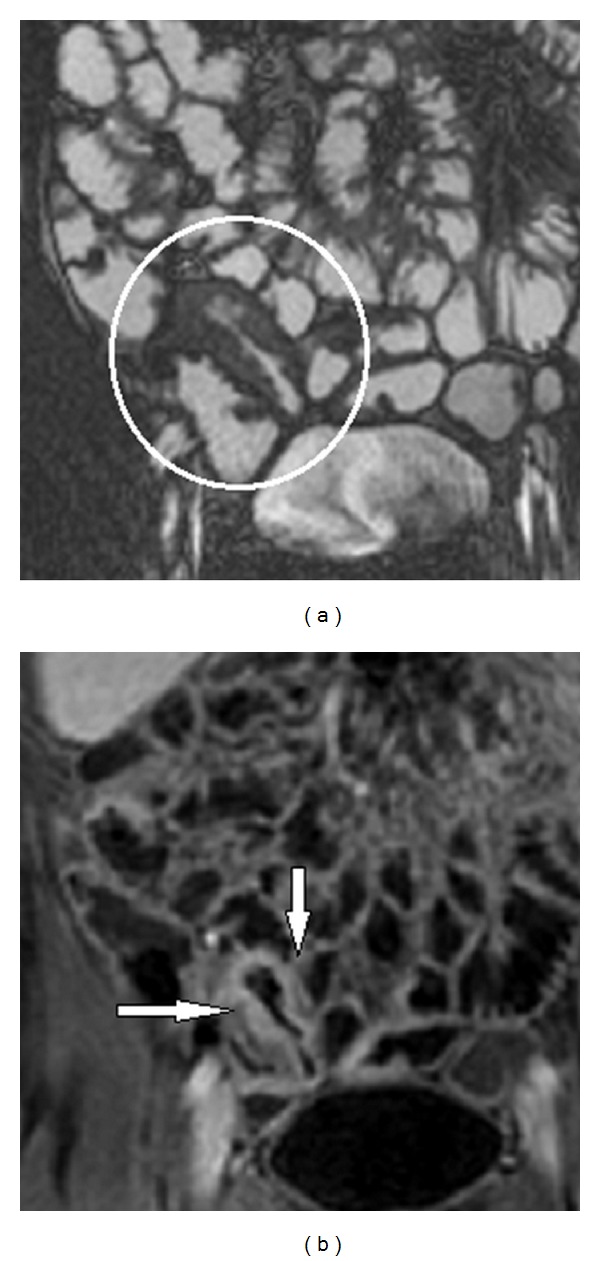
(a) HASTE sequence: mild inflammatory activity; distal ileum involved by disease with inhomogeneous wall thickening (white circle) (b) T1-weighted sequence (VIBE): postcontrast homogenous wall enhancement compliant with fibrotic evolution and reduced inflammatory activity (white arrows).
